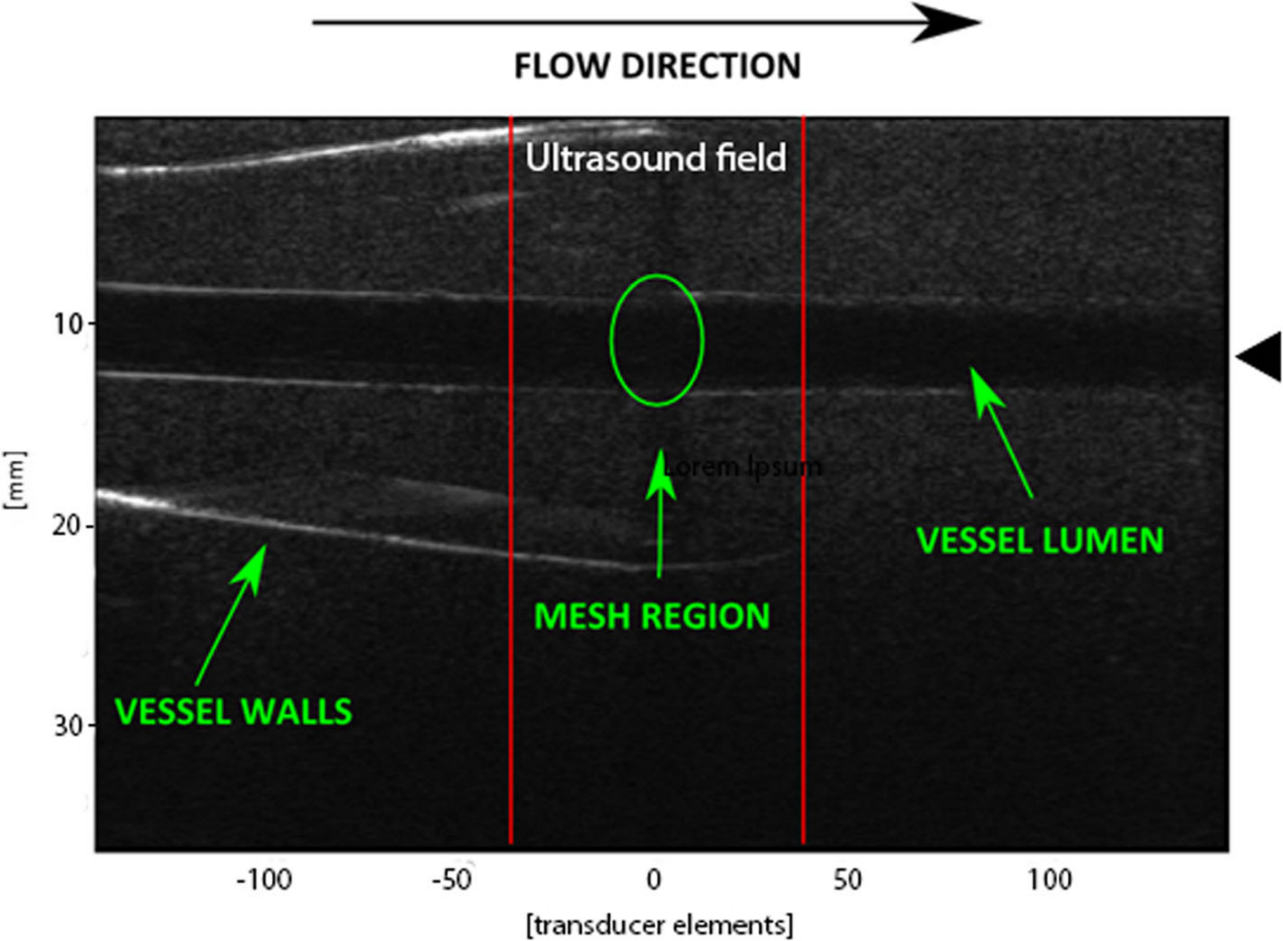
The ultrasound field using the L12–5 50 mm transducer
